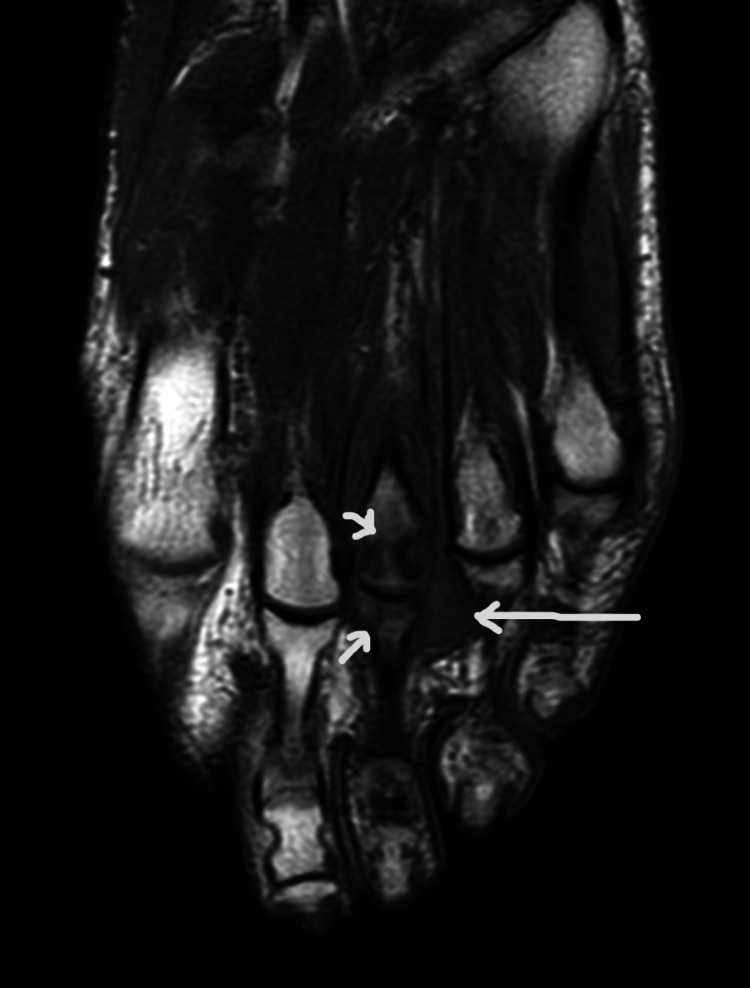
T1-weighted long-axis left-foot magnetic resonance imaging showing marked bone edema of the third metatarsal bone and the third proximal phalanx, and iso-signal widening of the third intermetatarsal space.
